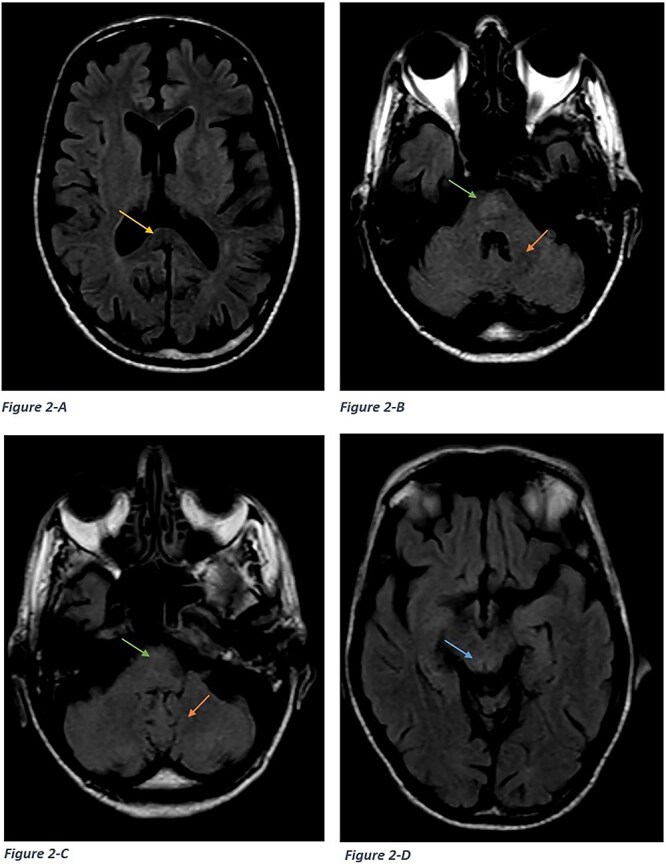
Follow up MRI done five days after cessation of the drug showed significant resolution of the lesions in the splenium of the corpus callosum (a yellow arrow), dorsal pons (B-C), bilateral dentate nuclei (C), and tectum (D).
